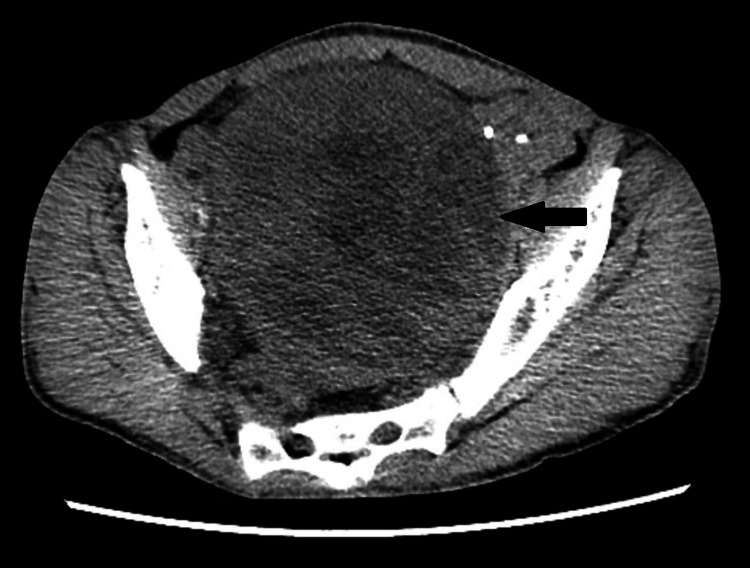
A CT scan of the pelvis (axial view) The black arrow points to the mass.
